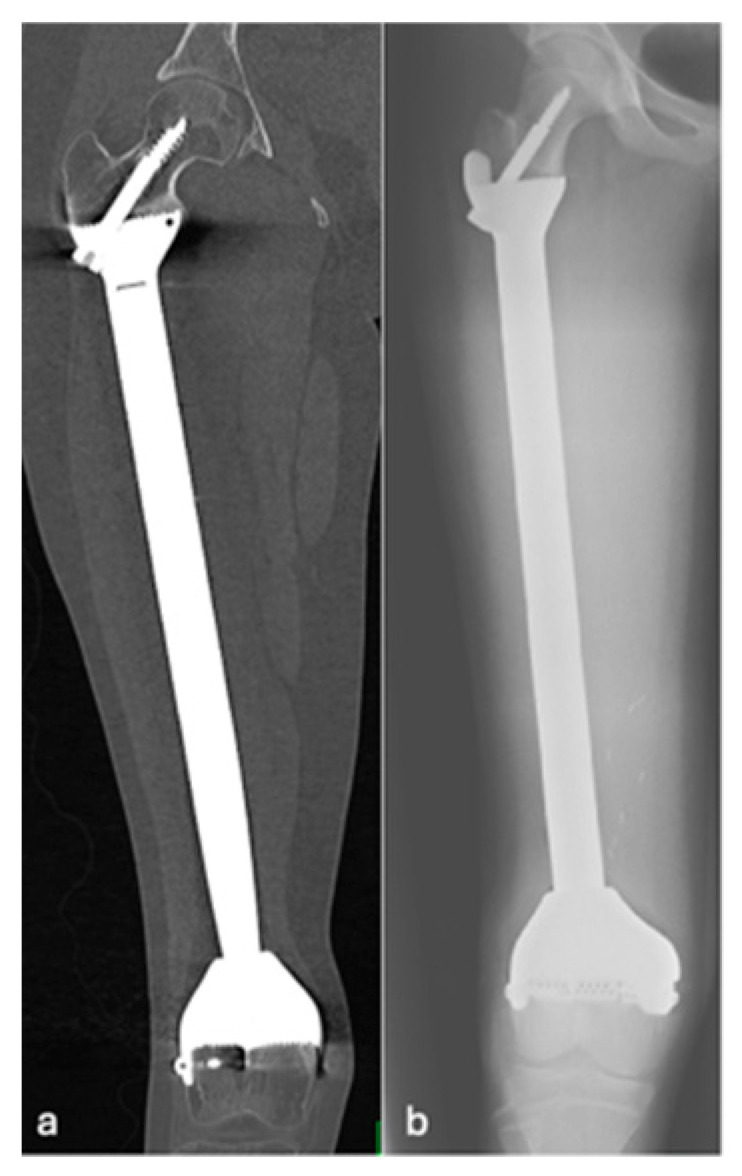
Coronal CT (a) and AP radiographs (b) showing grade 4 loosening of EPR with lucency around the proximal screw.
